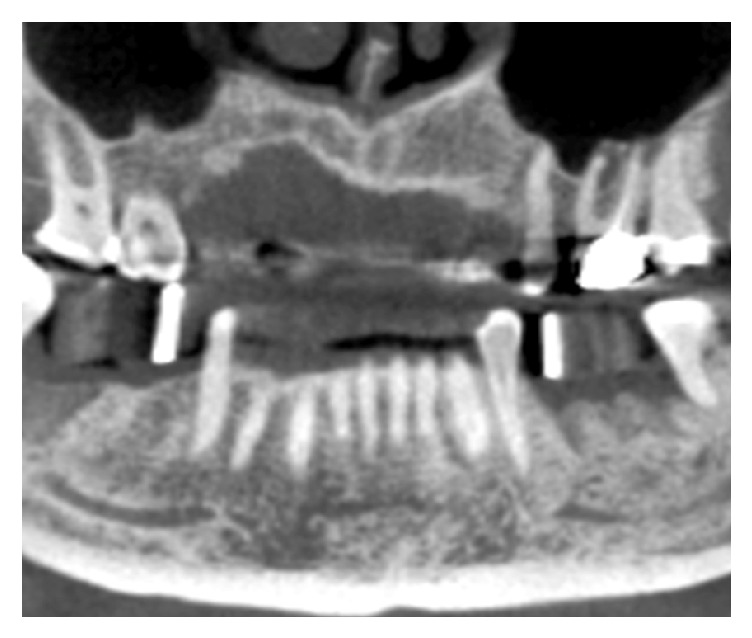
A panoramic view from CT with the radiological template in situ.
